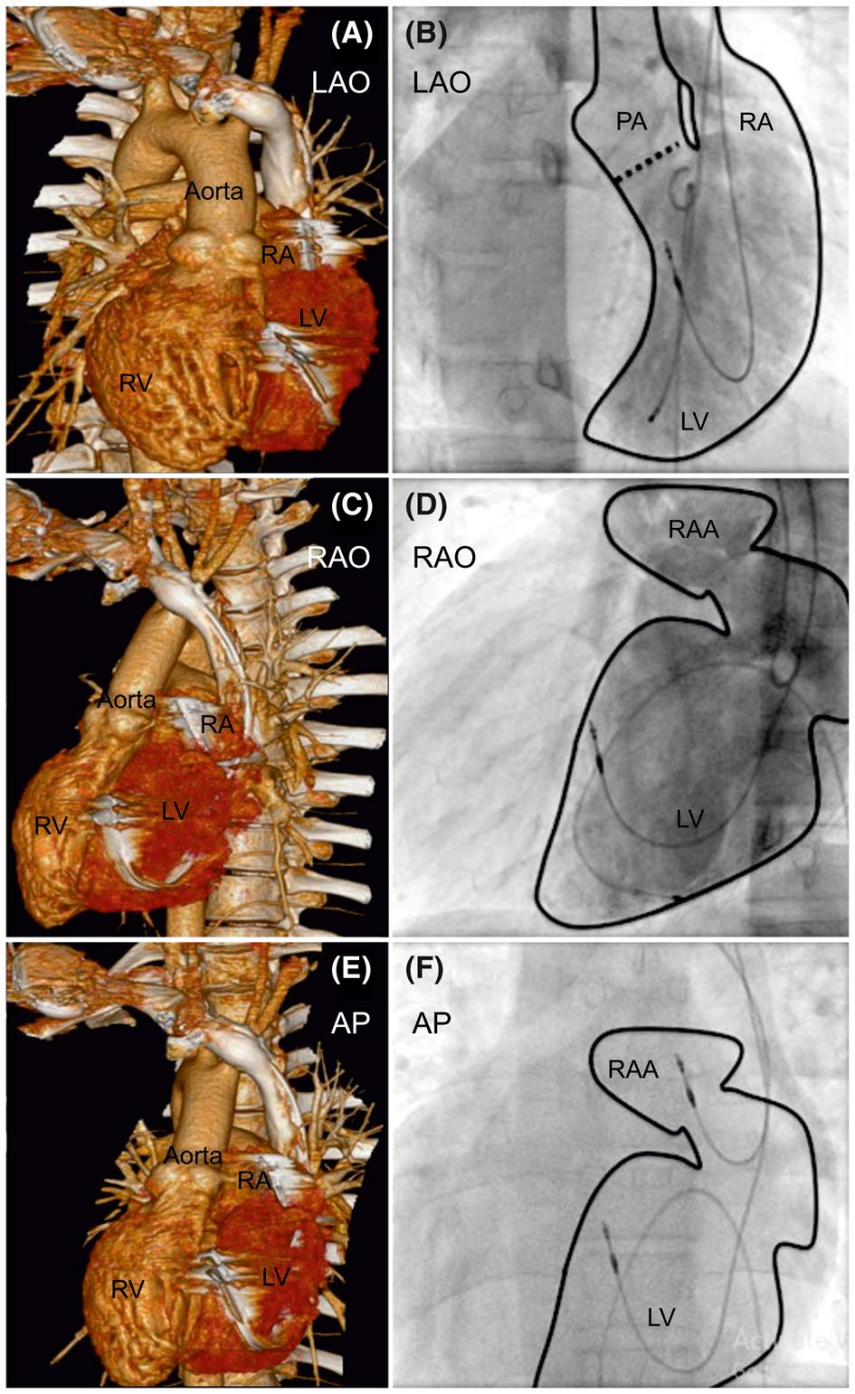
Computed tomographic reconstruction and angiogram of the dual‐chamber pacing system in a patient with situs inversus, dextrocardia, and corrected transposition of the great arteries. The left panels show computed tomographic three‐dimensional reconstructions of the heart. (A) Left anterior oblique, (C) Right anterior oblique, and (E) Anteroposterior views with the pacing lead tip position. Right panels (B), (D), and (F) show procedural angiographic images in the same views as (A), (C), and (E), showing ventricular lead position in the mid‐septal area in the morphological left ventricle, as confirmed by cardiac computed tomography. AP, anteroposterior; LAO, left anterior oblique; LV, left ventricle; RA, right atrium; RV, right ventricle; RAA, right atrial appendage; RAO, right anterior oblique; PA, pulmonary artery
